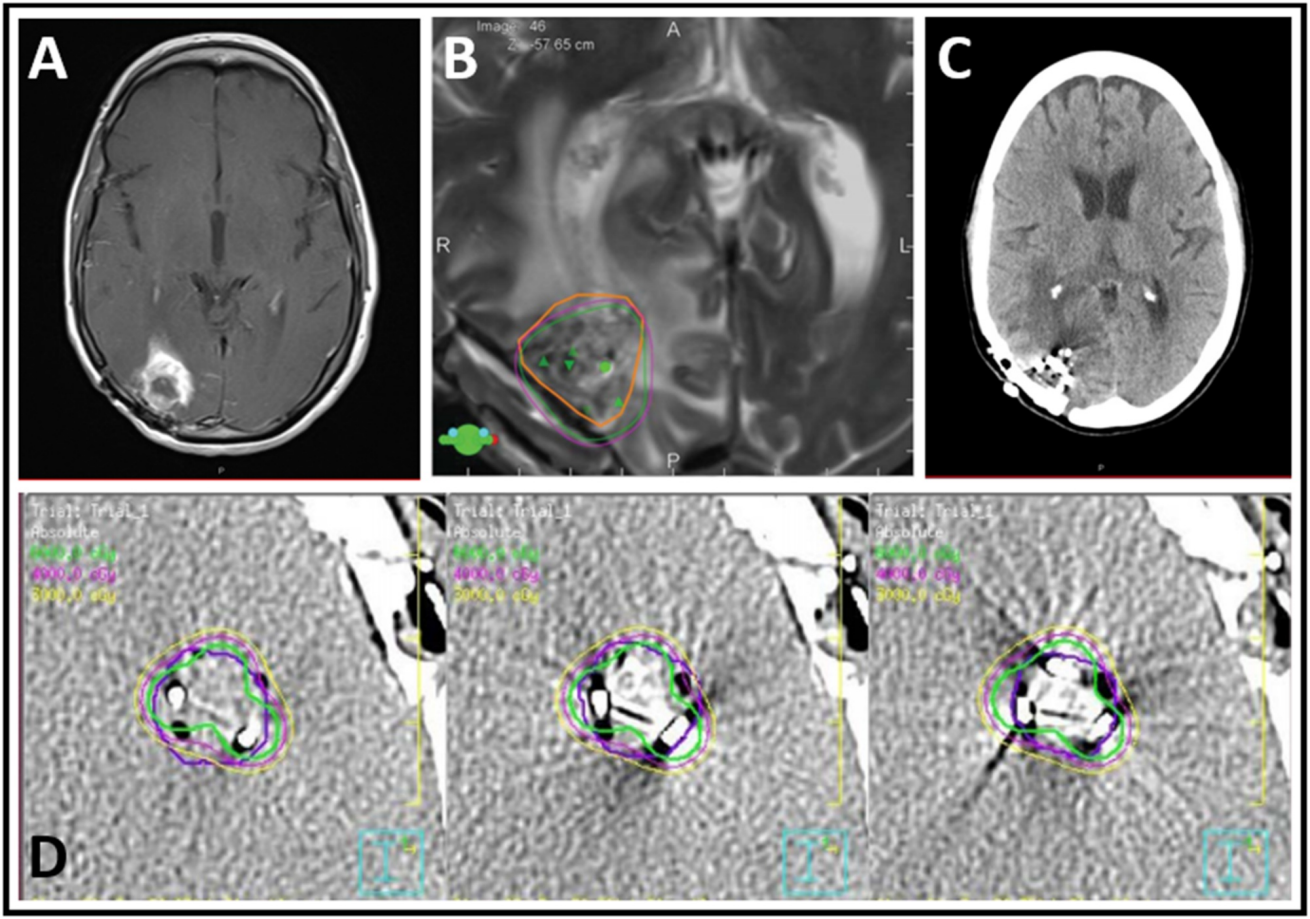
Series of images depicting preoperative, planning, and postoperative scans in a patient treated with Cs-131 brachytherapy. Preoperative T1 post-contrast magnetic resonance imaging (MRI) (A) depicts a 2.6 cm occipital lesion while post-operative T2 MRI dosimetry (B) shows the 60 Gy (purple) and 72 Gy (green) isodose lines overlayed on the planned target volume (orange). Post-operative computed tomography (CT) (C) confirmed seed placement with Leblanc dosimetry (D).
